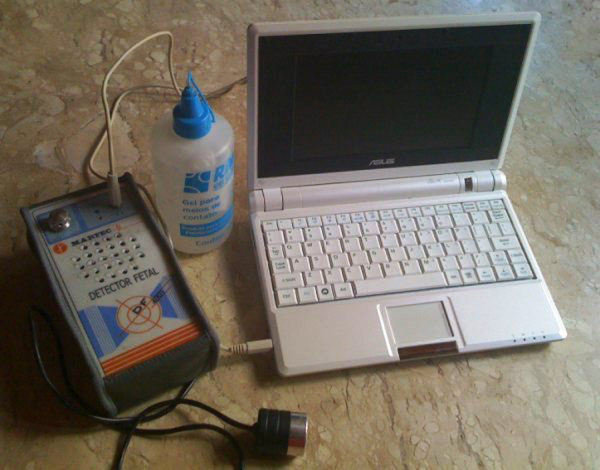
Ultrasonic detector (portable), DF-4001, Martec.
